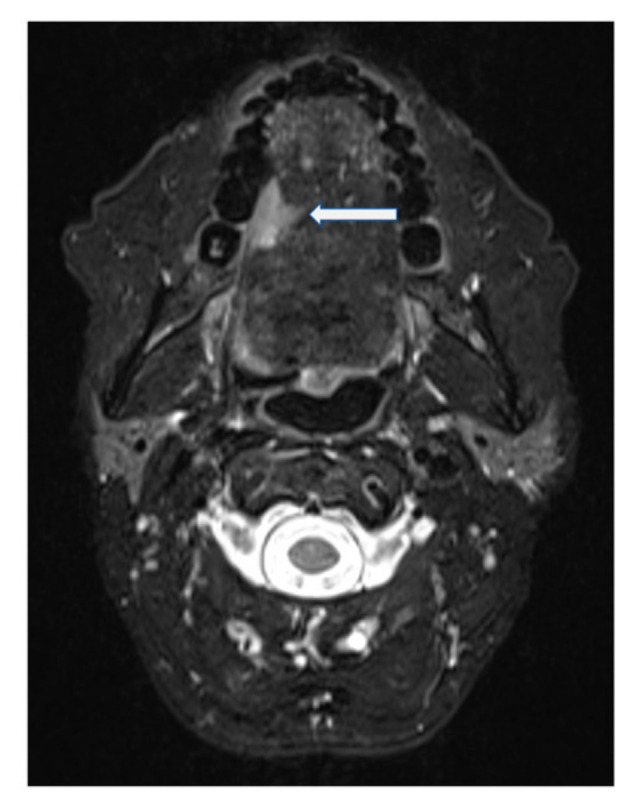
T2-weighted fat-suppressed, contrast-enhanced MRI of Case 2 in the axial plane. Squamous cell carcinoma indicated by arrow.
